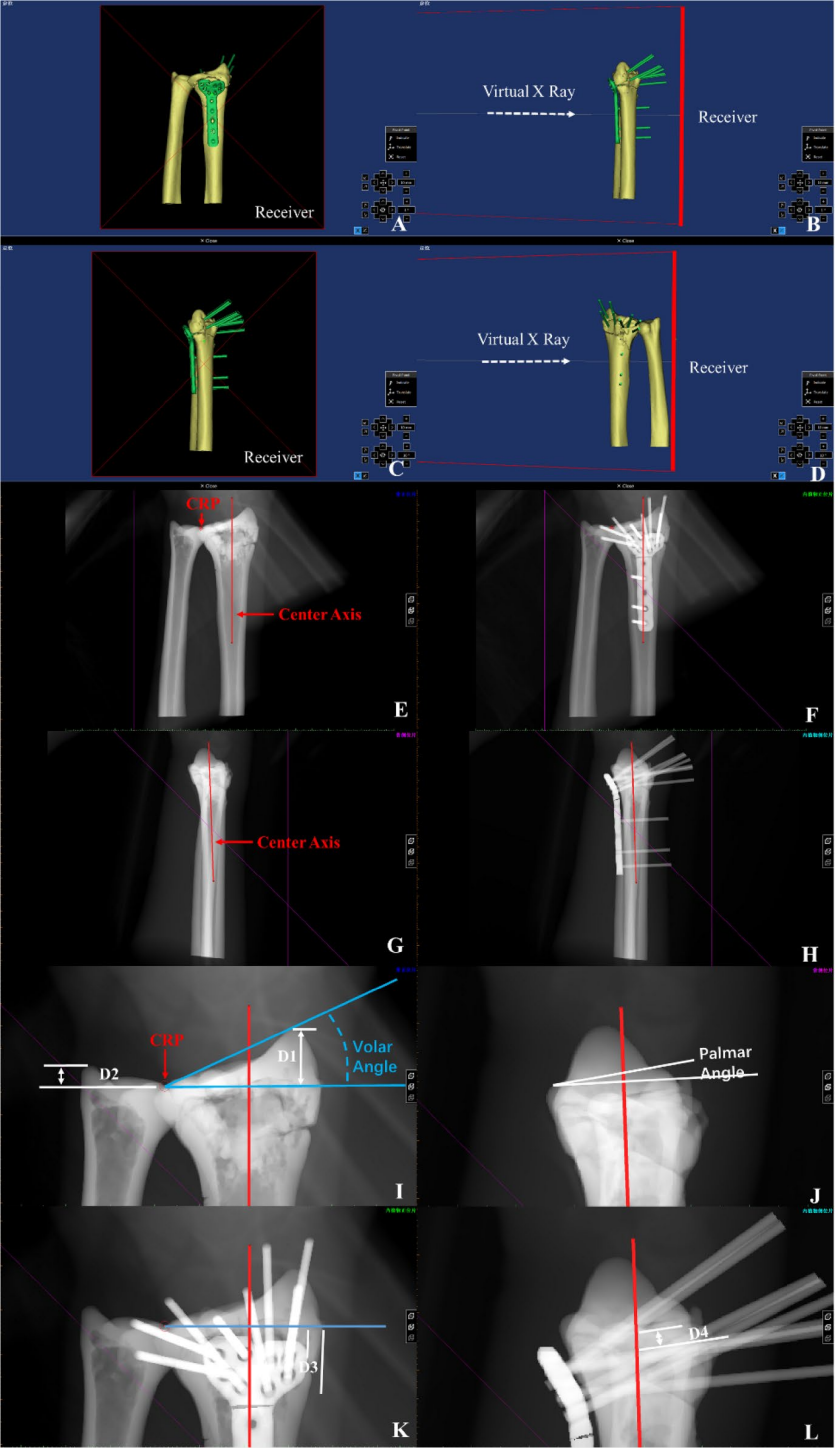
a and b The typical frontal imaging process, c and d the typical lateral imaging process, e and f the typical frontal images, g and h the typical lateral images, and i–l virtual X-ray measurement of different parameters
